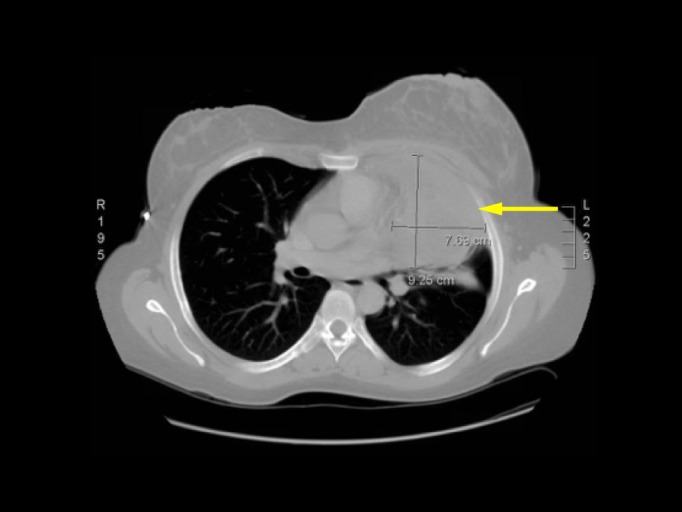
Computed tomography (CT) of the chest. A CT of the chest with contrast revealed a 7.69 cm x 9.25 cm anterior mediastinal mass with central calcification and extension into the anterior left chest abutting the pleura (yellow arrow).
